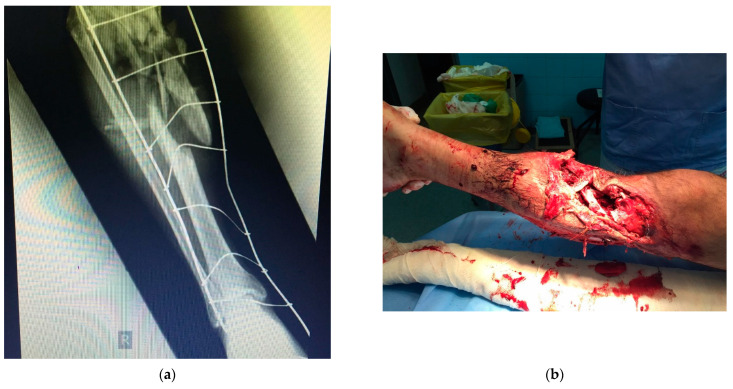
Open multifragmented fracture of the lower leg. Bone graft was applied. (a) radiography image of the fracture; (b) real image of the complex fracture before the surgical procedure.
